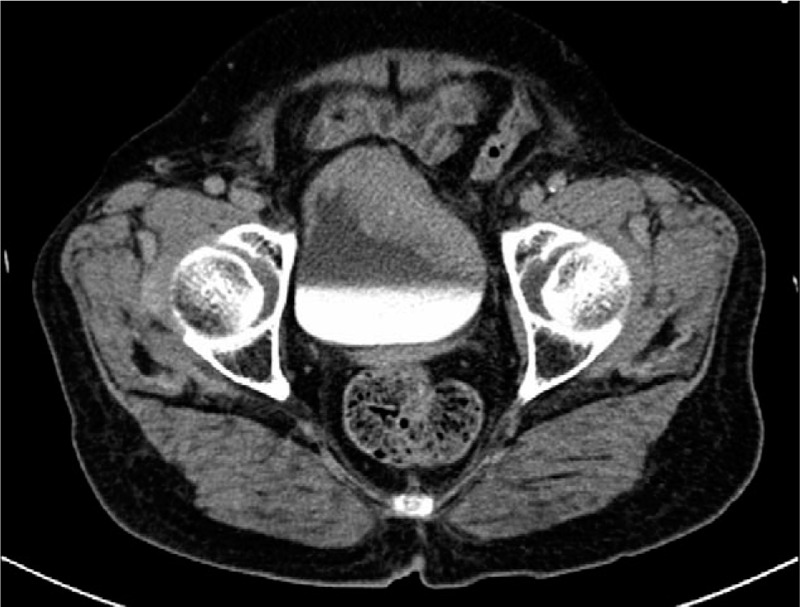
CT revealed extensive tumors in the anterior wall of the bladder.
